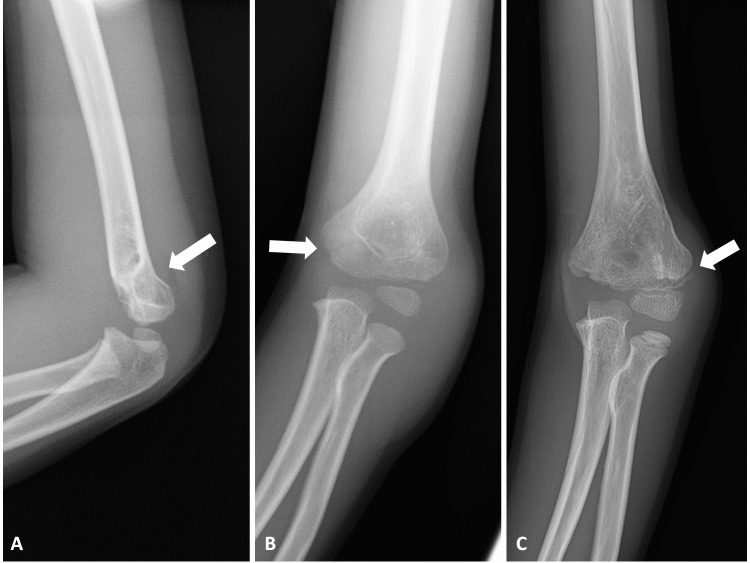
Plain radiography of the serial fractures at the left elbow. (A) The first fracture at the age of four years. (B) The second fracture at the age of five years. (C) The third fracture at the age of eight years. (Arrows: fracture sites).
